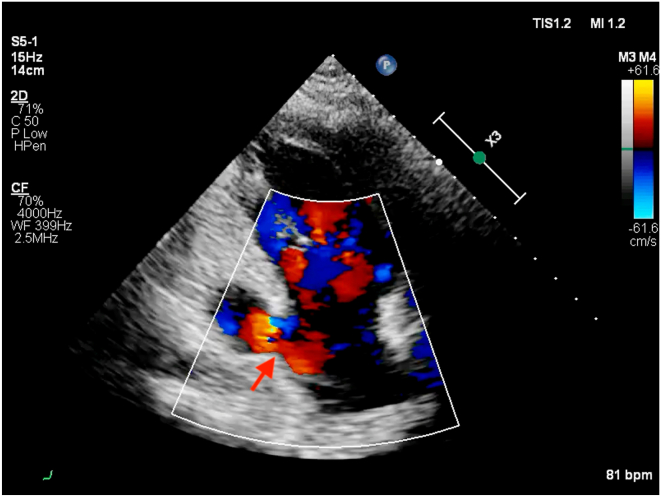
Two-dimensional TTE, apical 2-chamber systolic view with color-flow Doppler, demonstrates the dilated CS with a deficient LA floor *(star)* and laminar flow connecting these chambers *(arrow)*.
